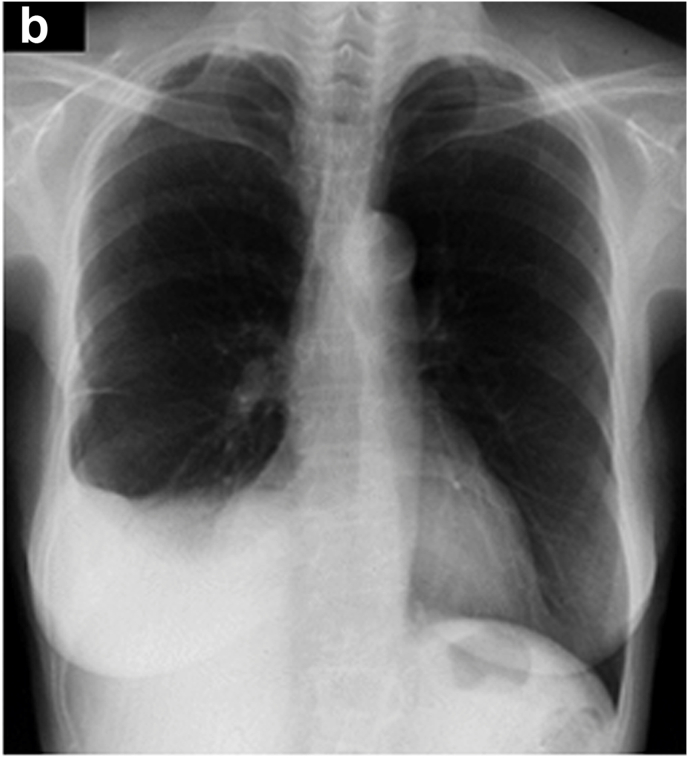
Chest radiographs Blunting of the right costophrenic angle has progressed, and a mass is observed in the thoracic wall.
